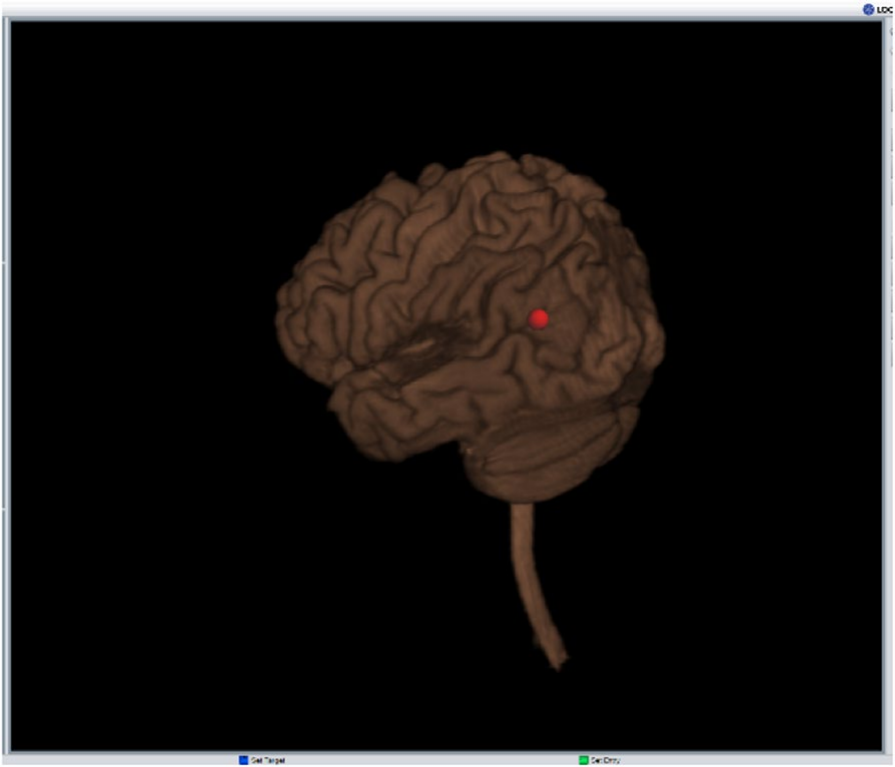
Example of target electrode location. Subjects’ heads are co-registered with MR images in a 3-D space to identify target location for the tDCS stimulation site. The red dot denotes the location of the target tDCS electrode
